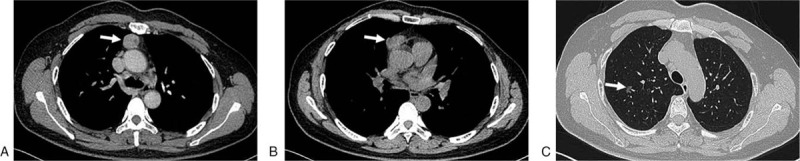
Thoracic computed tomography examination. (A) A mass in the anterior superior mediastinum (arrow). (B) A mass in the anterior mediastinum near the right pericardium (arrow). (C) A ground-glass opacity, about 1.0 cm in diameter, in the right superior lobe of the lung (arrow).
